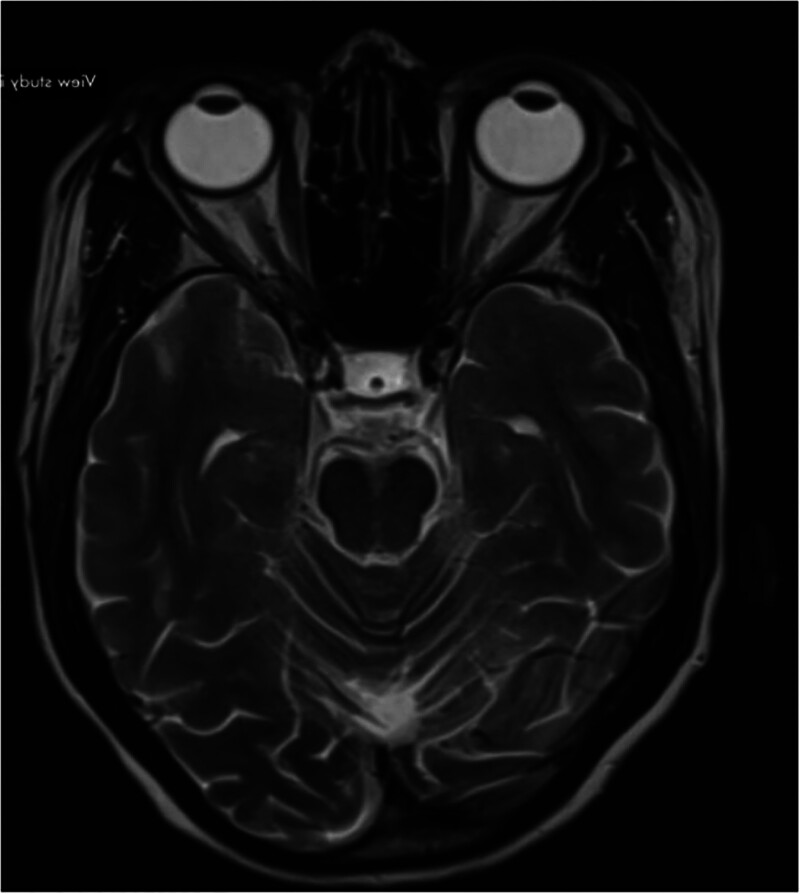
Axial T2W MRI image shows empty sella with a small T2 dot of the infundibulum (shown by white arrow).
